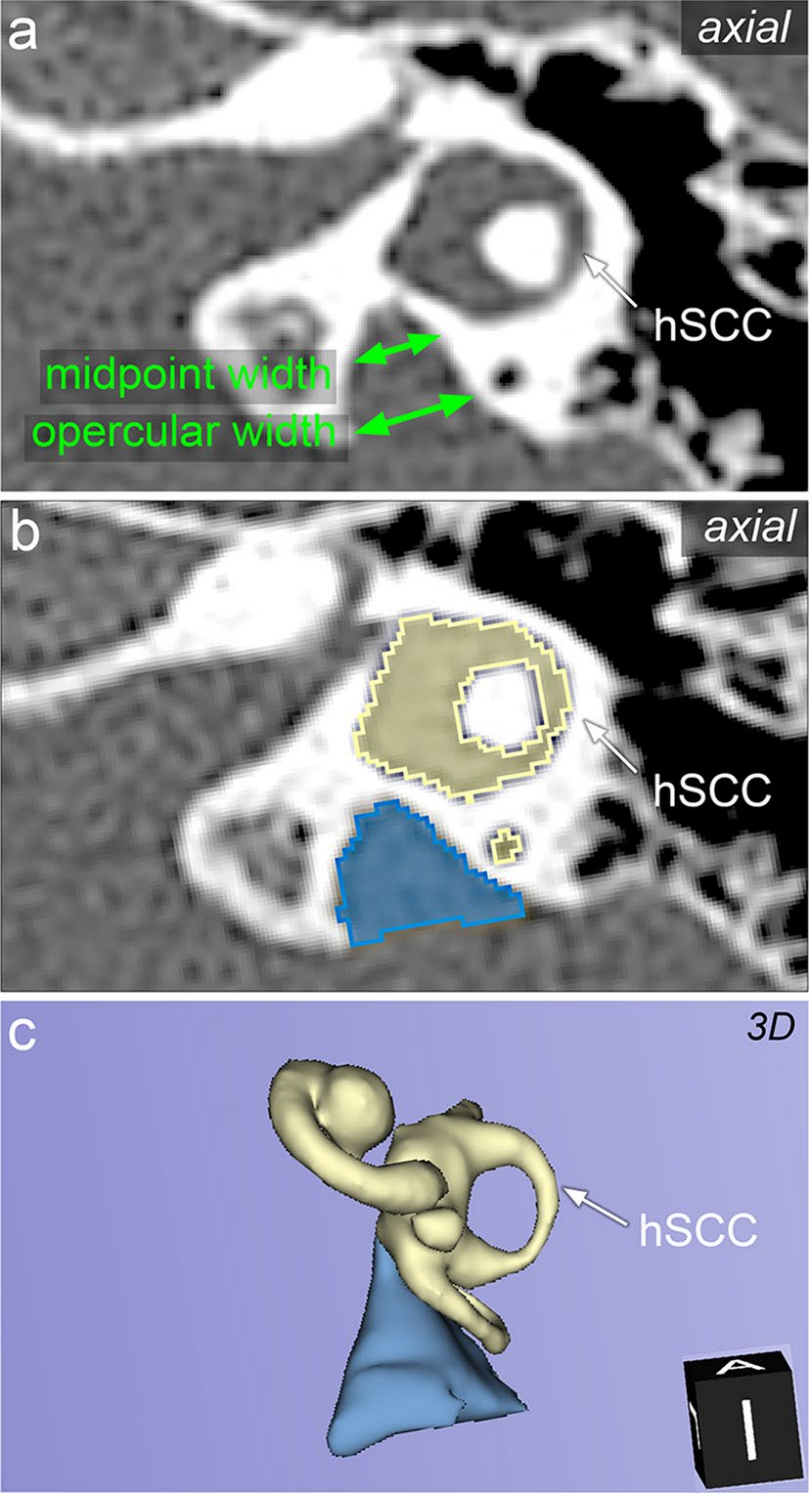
Exemplary measurements of the vestibular aqueduct (VA) width and 3D reconstruction in a temporal bone with an enlarged VA (EVA). a Two-dimensional measurements according to the Cincinatti criteria at the midpoint (upper green arrow) and the operculum (lower green arrow) of the VA in an axial high-resolution computed tomography (HRCT). b Segmentation of the 3D reconstruction from the axial HRCT. Yellow: segmentation of the bony labyrinth. Blue surface: segmentation of the VA. c Three-dimensional reconstruction of the bony labyrinth (yellow) and the EVA (blue). *hSCC*, horizontal semicircular canal
